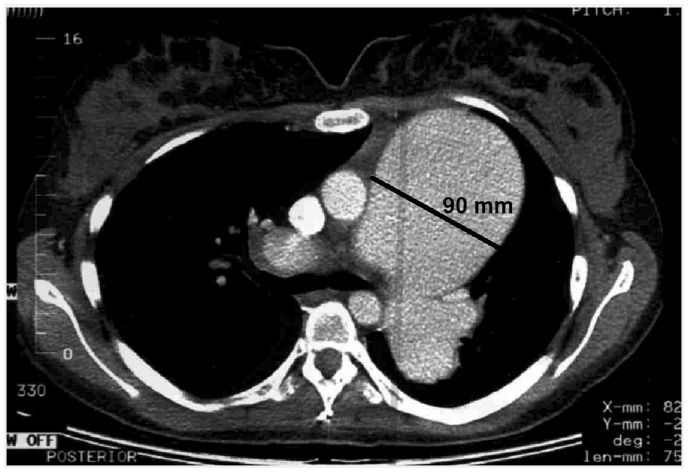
CT scan demonstrating an aneurysm of the pulmonary artery.
